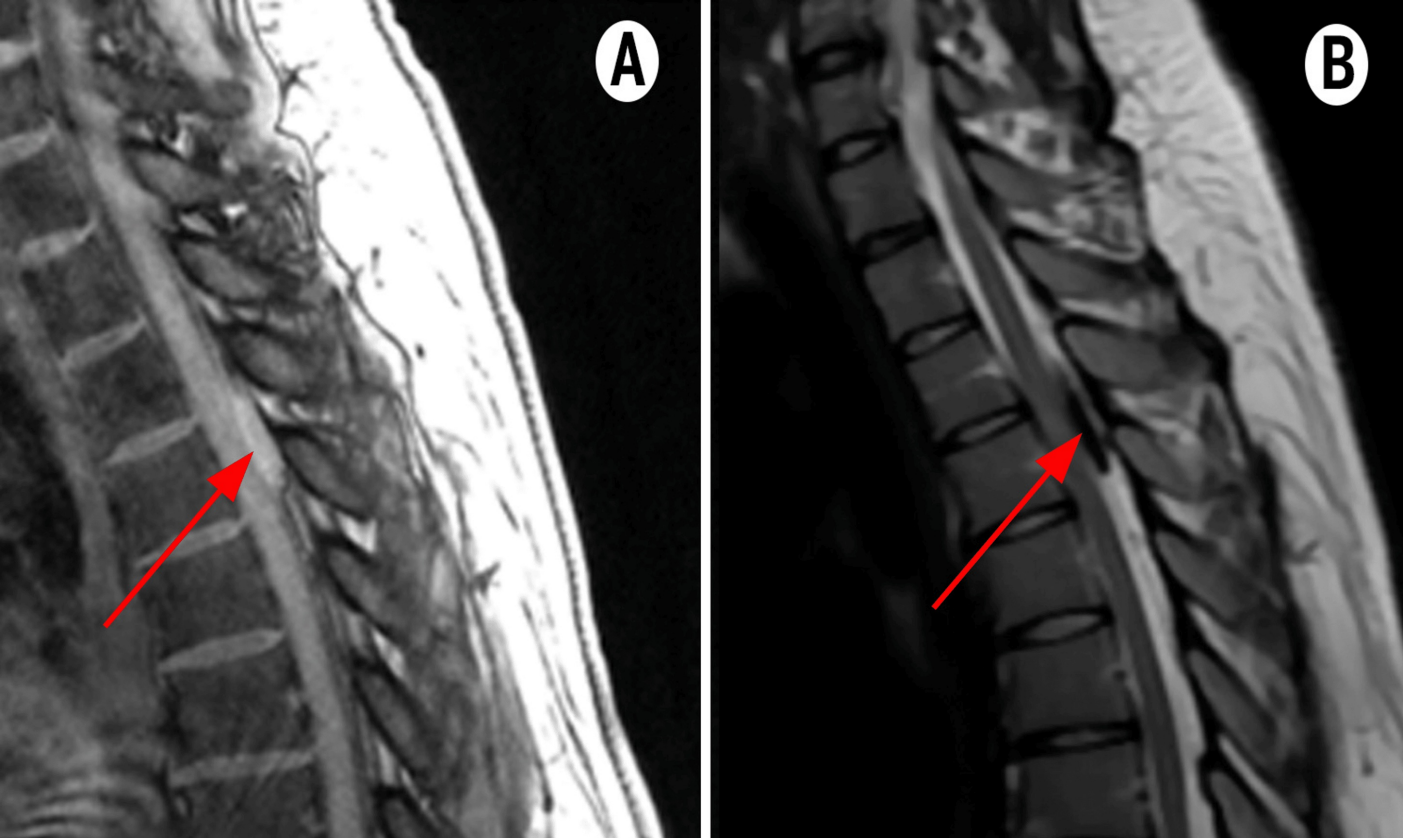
Thoracic spine MRI. Thoracic spine MRI showing an extramedullary tissue process centered on the meninges at the D3-D4 level, measuring 7 × 12 × 28 mm (red arrow). It appears isointense on T1 and T1 fat saturation (A) and hypointense on T2 (B).
